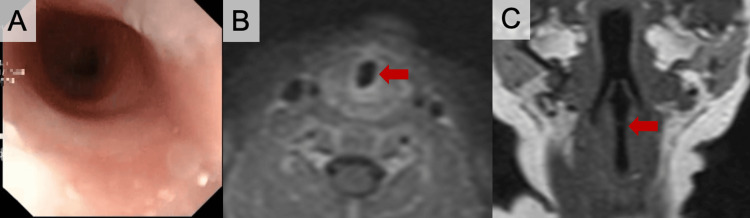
Posttreatment Laryngoscopic and MRI Findings Showing Lesion Regression Follow-up laryngoscopy performed immediately after extubation (A) and MRI (B, C) reveal marked regression of the subglottic hemangioma with improvement in airway patency. Red arrows indicate the widened airway lumen. MRI, magnetic resonance imaging
